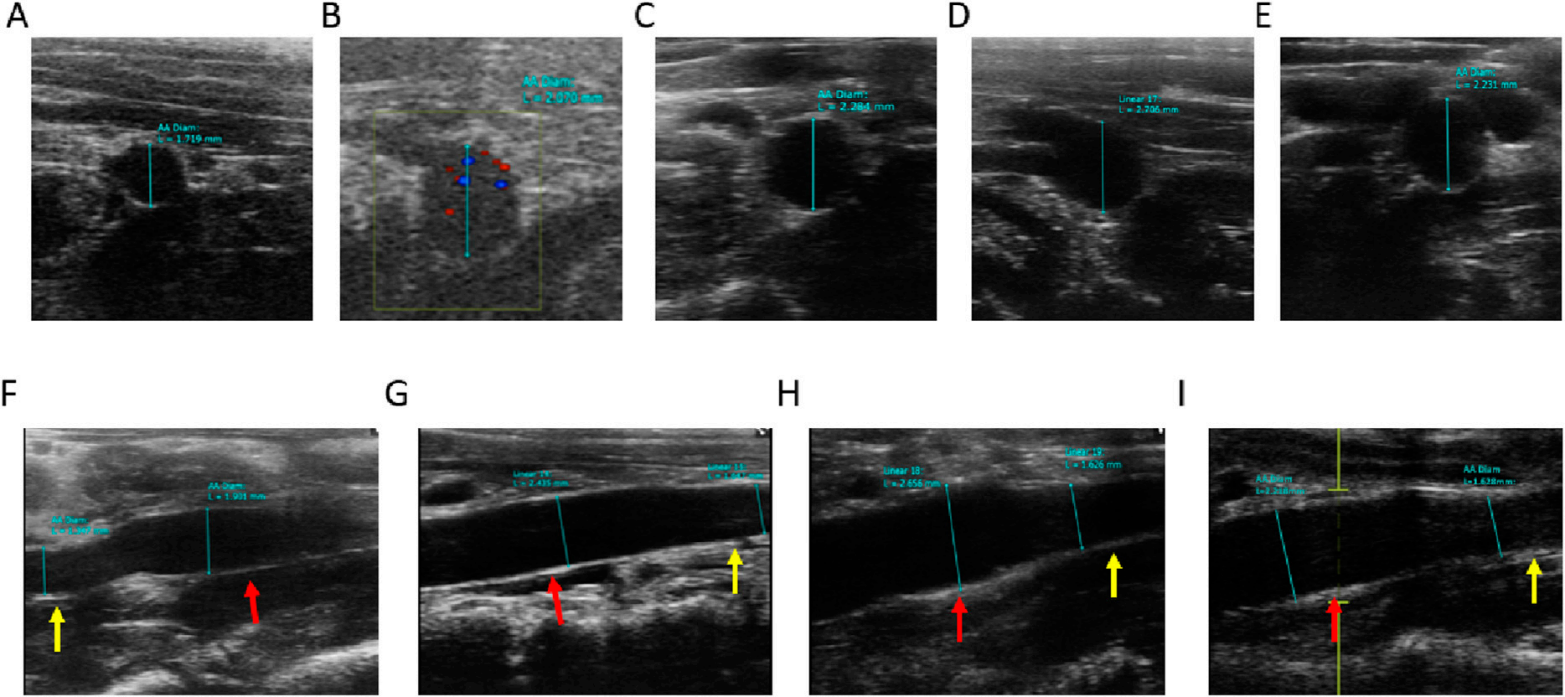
Ultrasound measurement of abdominal aortic vessel diameter, red arrows indicate dilated areas, yellow arrows indicate normal areas. (A) Control group short-axis ultrasound images. (B–E) are short-axis ultrasound images of AAA-1w, AAA-2w, AAA-3w, and AAA-4w, respectively. (F–I) are long-axis ultrasound images of AAA-1w, AAA-2w, AAA-3w, and AAA-4w, respectively.
